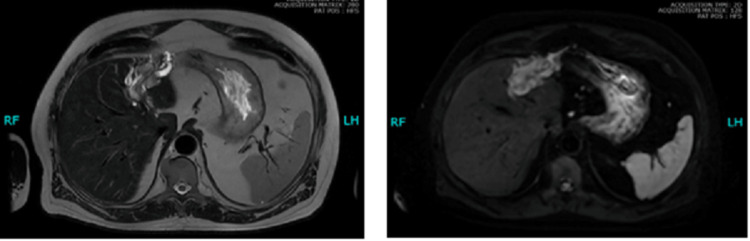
Contrast-enhanced MRI showing a focally enhancing lesion in the left liver lobe appearing isointense in the arterial phase (A) and showing diffuse restriction (B).
